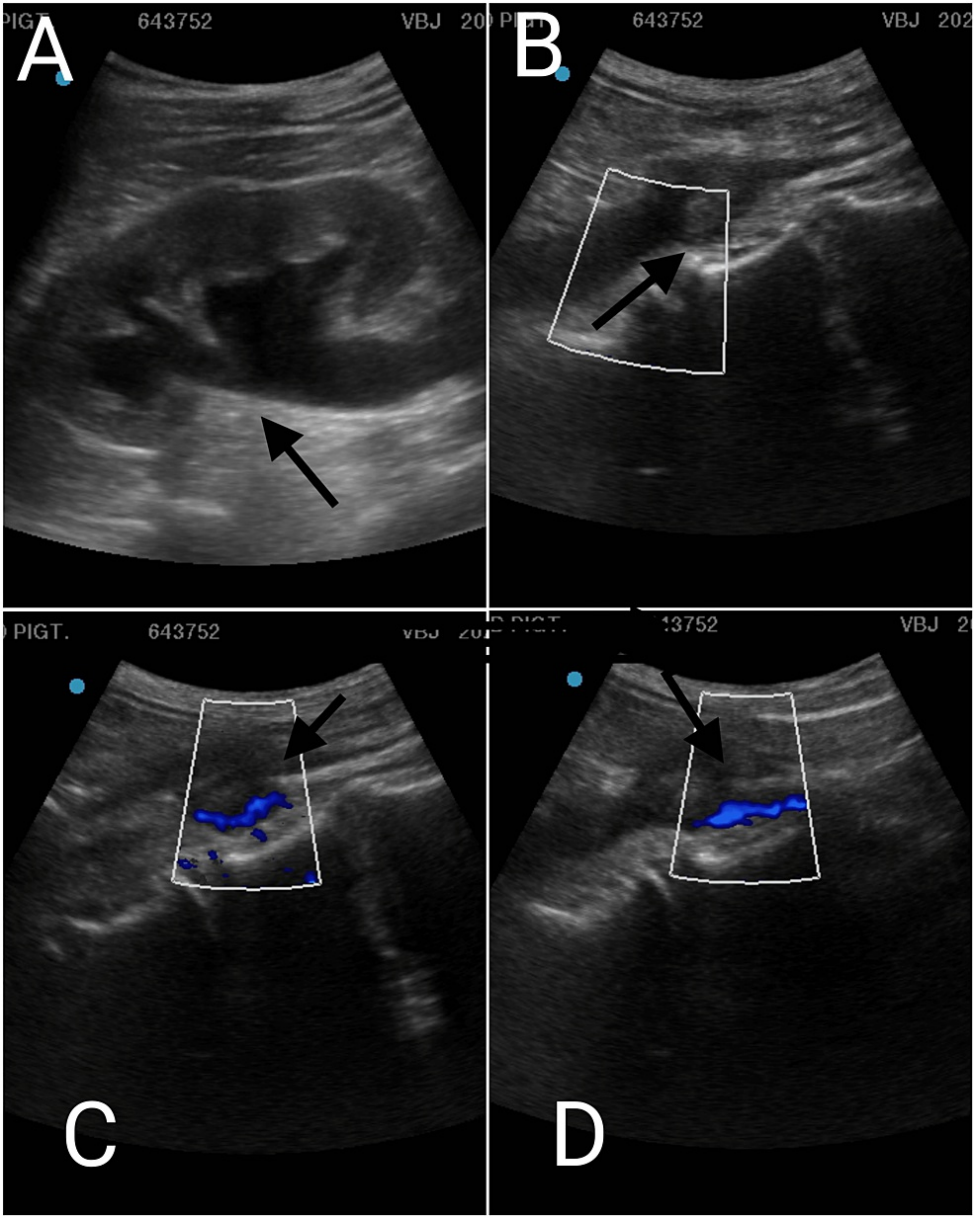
Doppler ultrasound of the patient (A) Ultrasound of abdomen showing hydronephrosis. (B) Color Doppler image showing ureter along with mass lesion. (C) Color Doppler image showing the upper one-third of ureter and mass with inferior vena cava. (D) Color Doppler image showing the mass compressing and infiltrating the lumen of the inferior vena cava.
